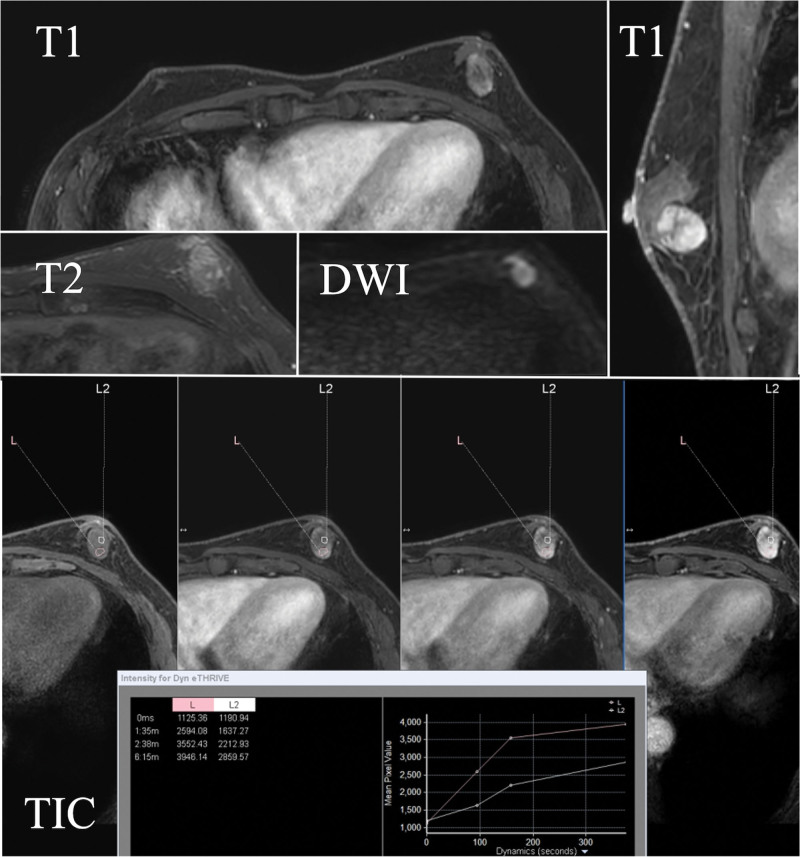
Magnetic resonance imaging: A well-defined and oval lesion was heterogeneously high signal intensity on T1WI and T2WI with time intensity curve of rapid-plateau pattern.
